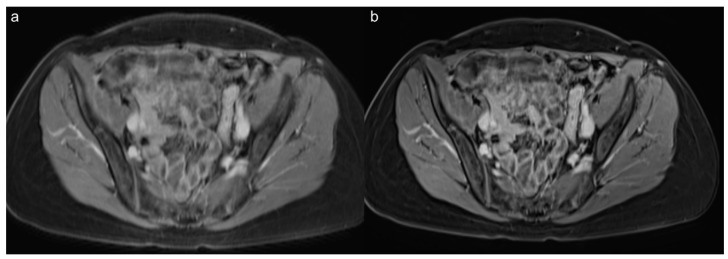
Follow-up abdominopelvic MRI in a 51-year-old male patient who had undergone Whipple surgery because of neuroendocrine neoplasia of the papilla VATERI. The postcontrast VIBE_SR_ images (b) show a distinctively better SNR and sharpness of the lymph nodes and the intestine than the VIBE_Std_ postcontrast VIBE images (a).
